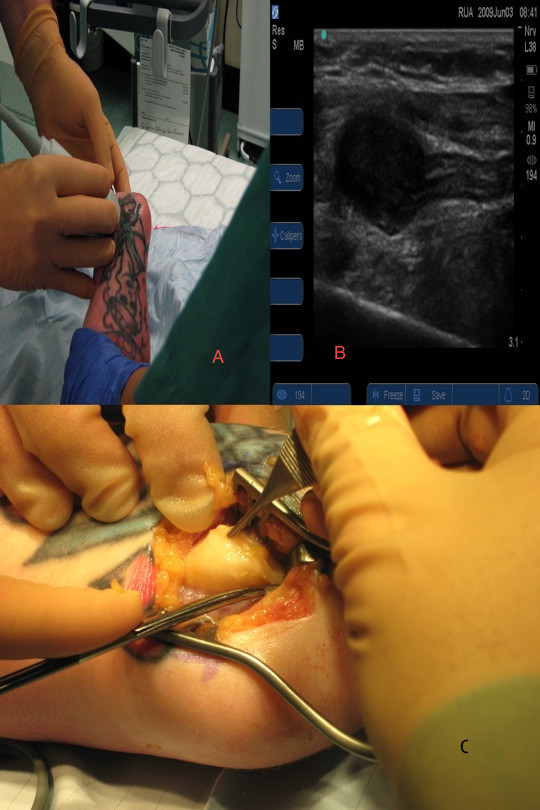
Localization of tumor and surgical excision of neuroma. [rvm1] (A) Ultrasonographic localization of tumour, (B) ultrasonographic images, (C) surgical excision of neuroma following ultrasonographic localisation.
